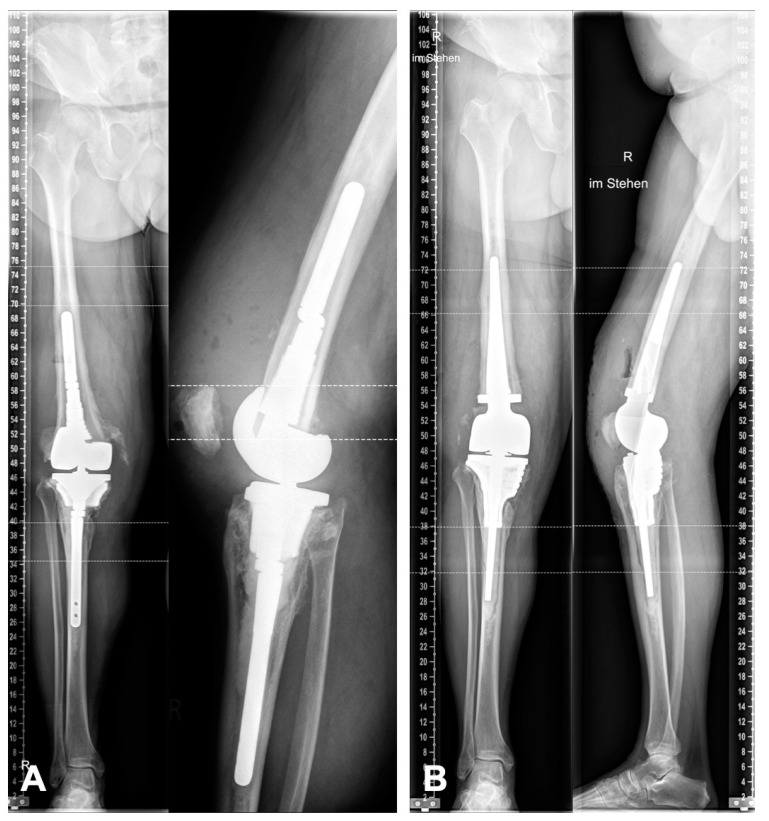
(A) Preoperative X-rays before explantation of the septic prosthesis. (B) Postoperative X-rays after reimplantation. The joint line and limb alignment are fully reconstructed. (R = right).
